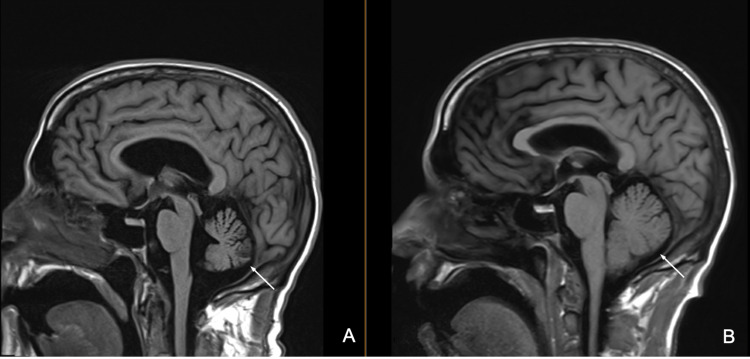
One-year follow-up MRI of the brain. Follow-up MRI one year after initial presentation showing progressive cerebellar atrophy (A) compared to previous imaging (B) with an improvement of hyperintensities.
